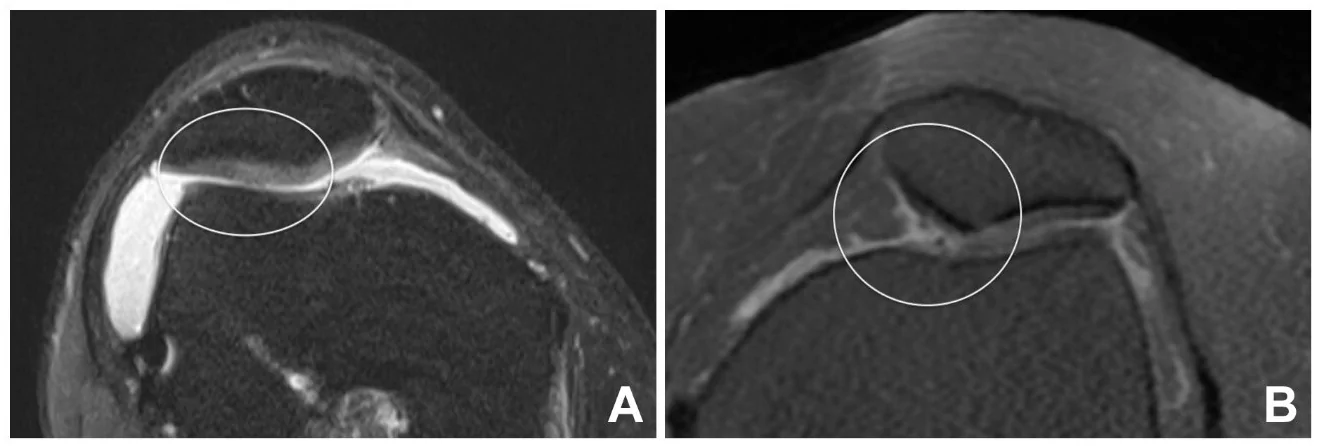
(A) Grade 1, (B) Grade 2 chondromalacia on axial MRI.
